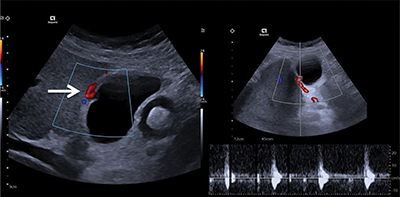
Doppler ultrasound showing a 5 mm anechoic micro-cavitary formation on the posterior wall of the gallbladder, presenting arterial flow, suggestive of a pseudo-aneurysm of the cystic artery.
